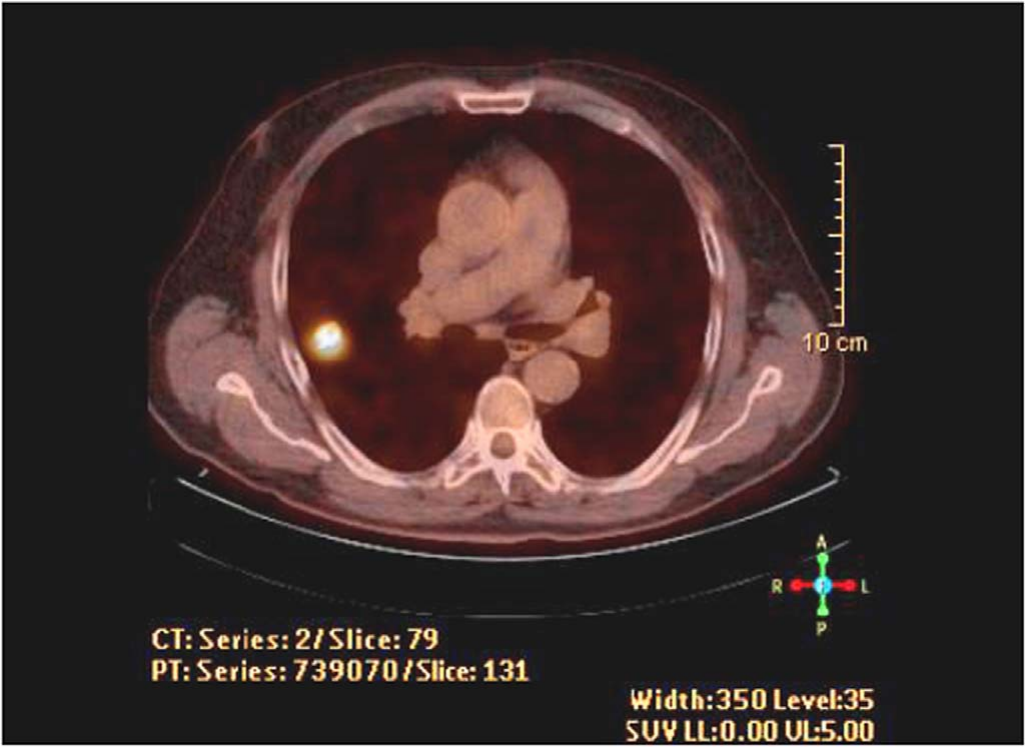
PET-CT scan of solitary malignant pulmonary nodule reflecting cancer growth as shining spot (SUVmax 5.0).
